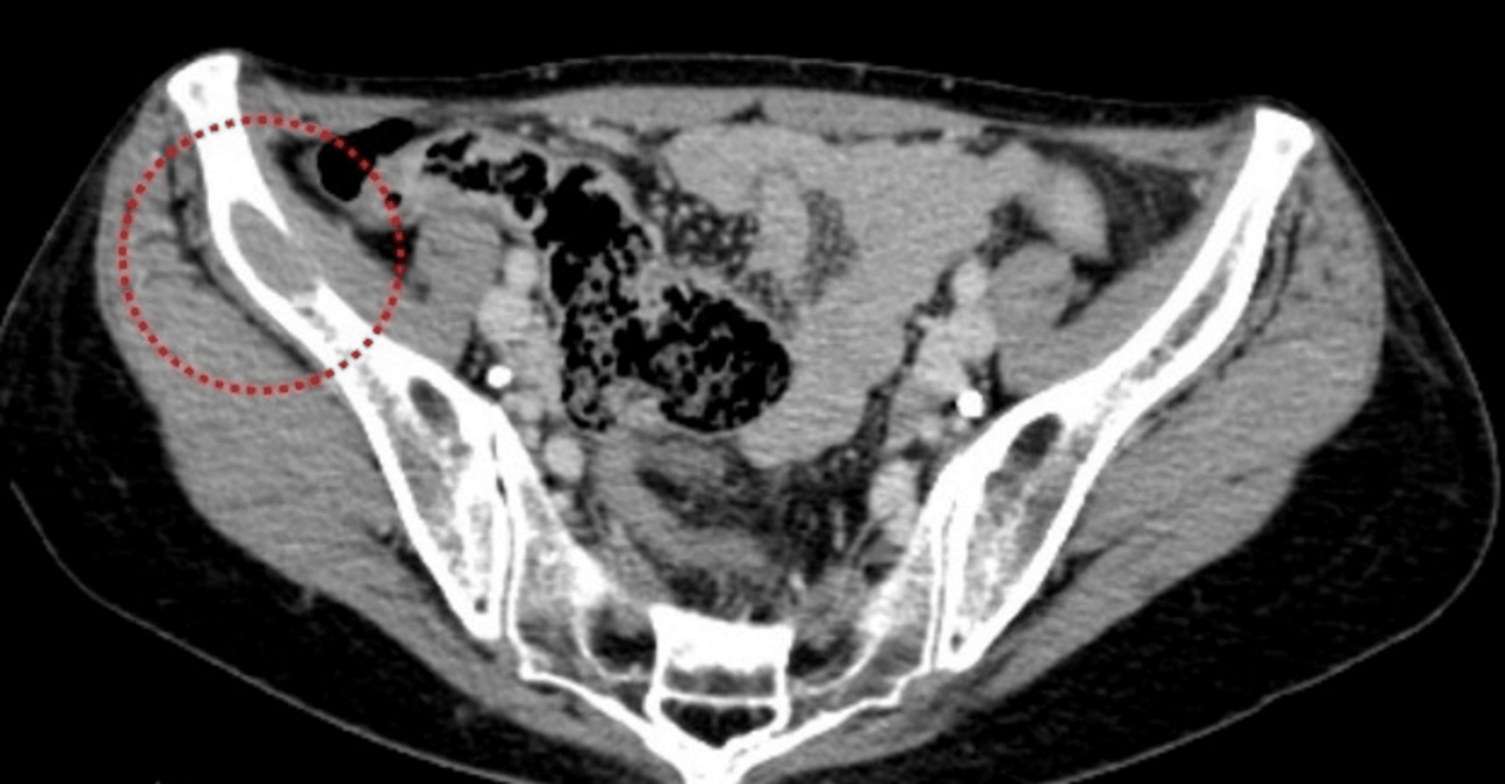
Retrospective review of the preoperative CT A low-density lesion (red circle) is visible in the iliac bone, suggesting possible metastatic involvement associated with the primary angiosarcoma.
